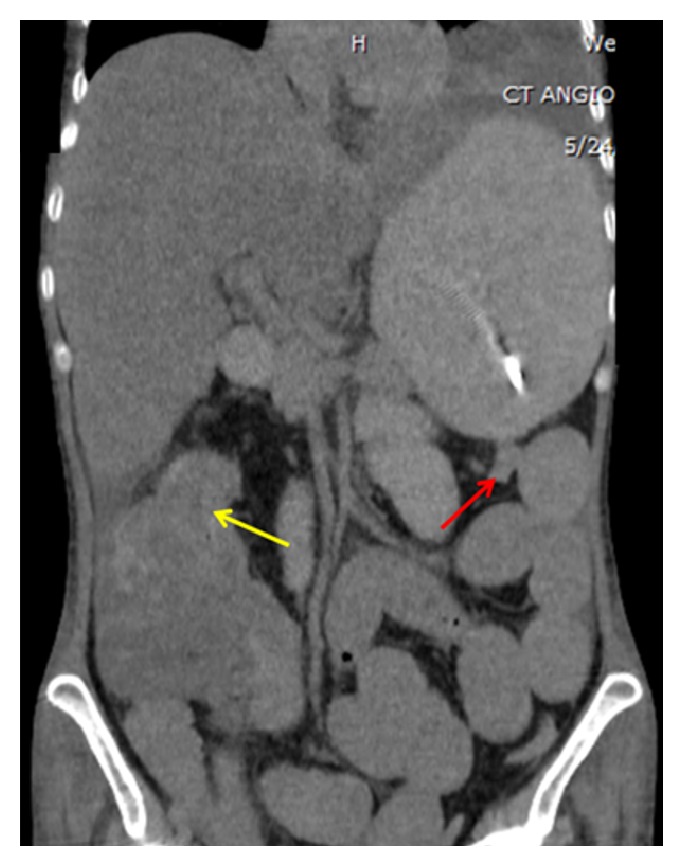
CT demonstrating distal luminal narrowing of bowel loops at both the splenic flexure (red arrow) and hepatic flexure (yellow arrow) with associated bowel wall thickening and edema.
